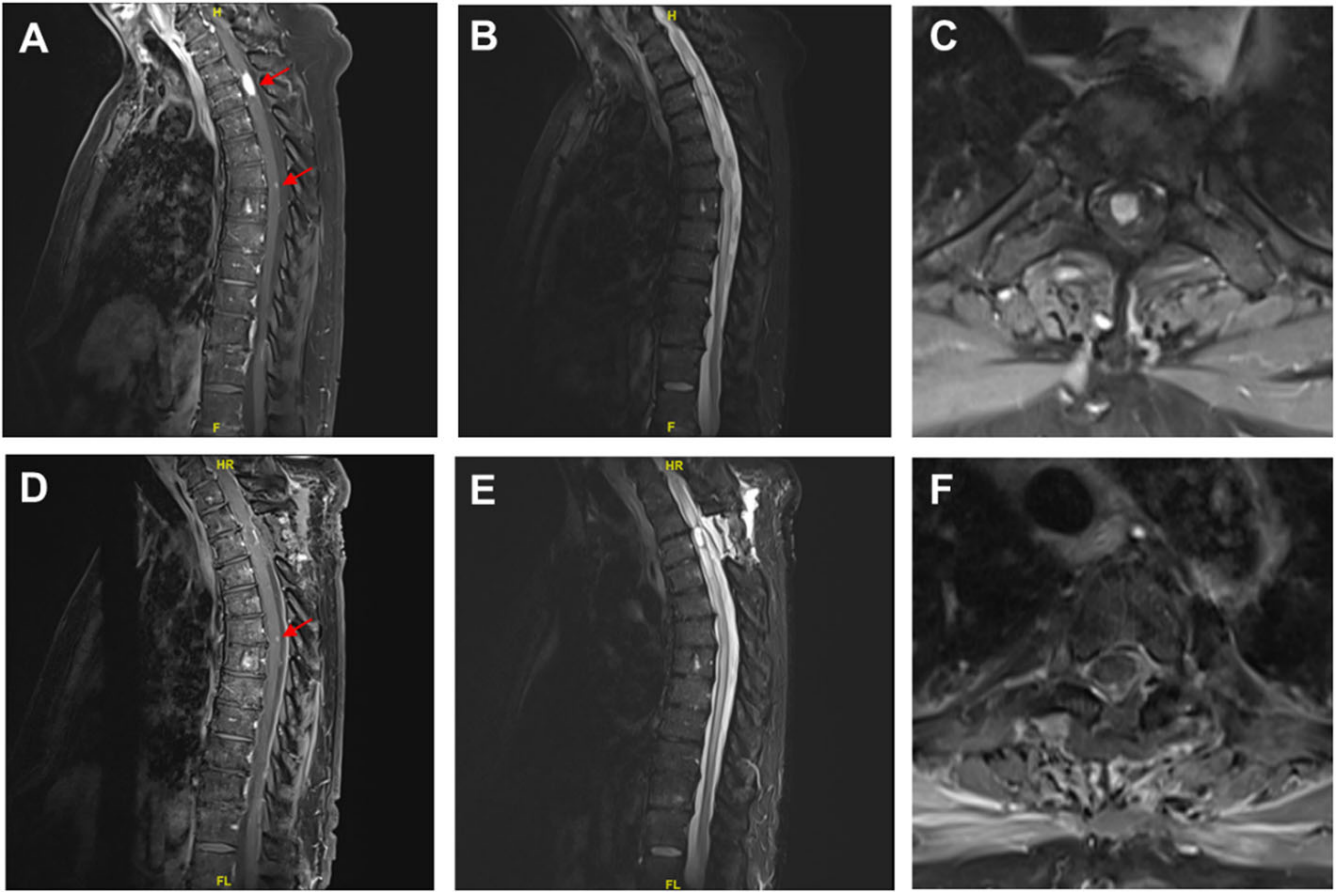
a–c Post-contrast sagittal T1-WI (T1-WI + contrast), T2-WI STIR, and axial T1-WI + contrast images showing intramedullary metastatic lesion (red arrows). Sagittal sequences show a dramatically enhancing lesion at the T2–T3 vertebral level and a second enhancing lesion in the dorsal cord at T6–T7. Axial sequences confirm the presence of the T2–T3 intramedullary spinal cord lesion. d–f Post-operative sequences showing complete resection of the T2–T3 lesion (red arrow). Expected post-surgical changes are seen with associated spinal cord edema. The T6–T7 lesion was not resected and remains identifiable on T1-WI + contrast magnetic resonance image. *STIR* short tau inversion recovery, *WI* weighted image
